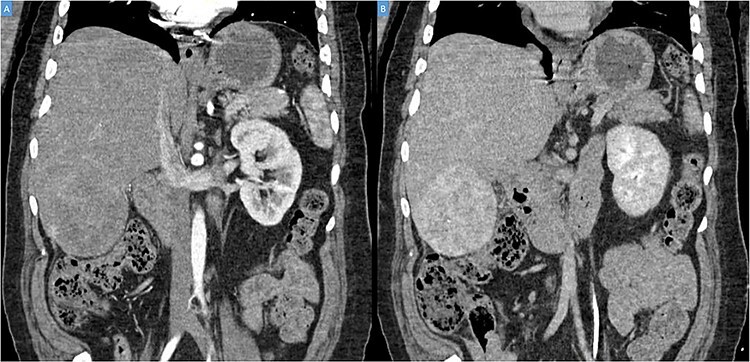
(A, B) Coronal computed tomography of the abdomen showing a large tumor on liver’s segments V–VI with heterogenous contrast enhancement.
